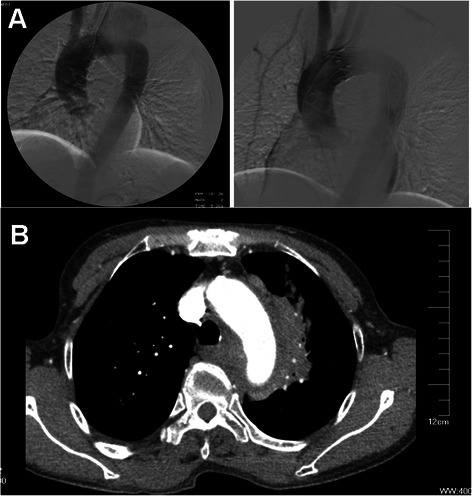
The determination of treatment effect by comparing. a. DSA angiography before intravascular graft exclusion for thoracic aortic pseudoaneurysm (Left) and angiography after operation (Right); b. Reexamination by Chest CT 6 days after operation
